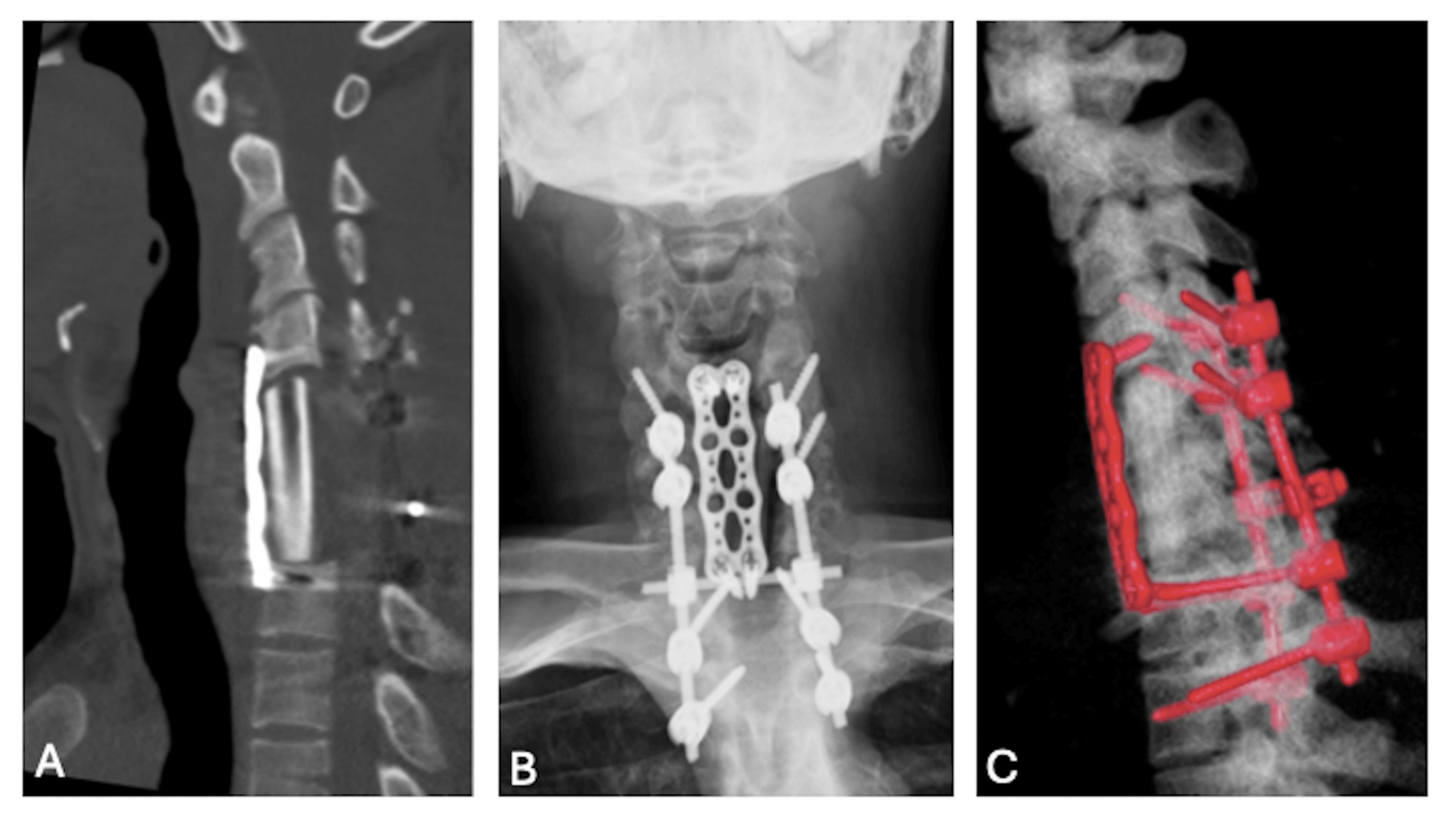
Postoperative images (A, C) Postoperative X-ray and three-dimensional reconstruction images showing the accurate positions into which the plates and screws were installed. (B) Postoperative bone window cervical sagittal CT showing fibular autograft placed in a proper position. CT: computed tomography
